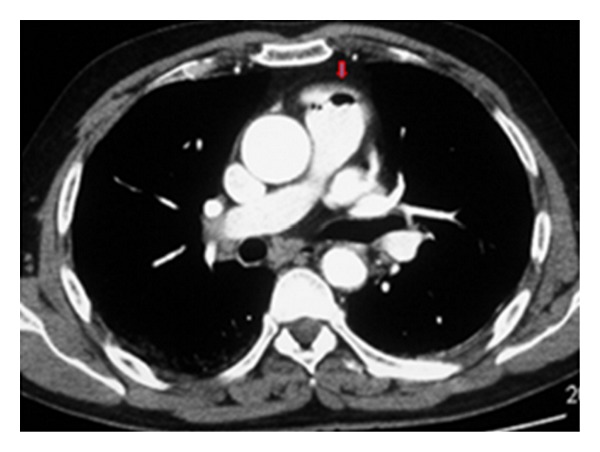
An axial CT scan shows air embolism with air in the pulmonary artery following the withdrawal of a central venous line (arrow).
